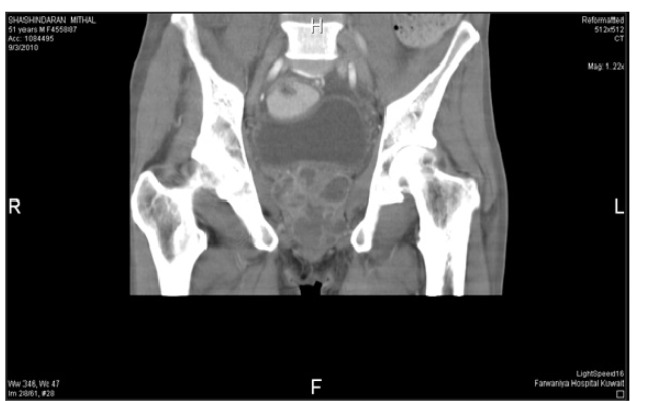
CT Scan Showing Multiple Diffuse Abscesses Within the Prostate
